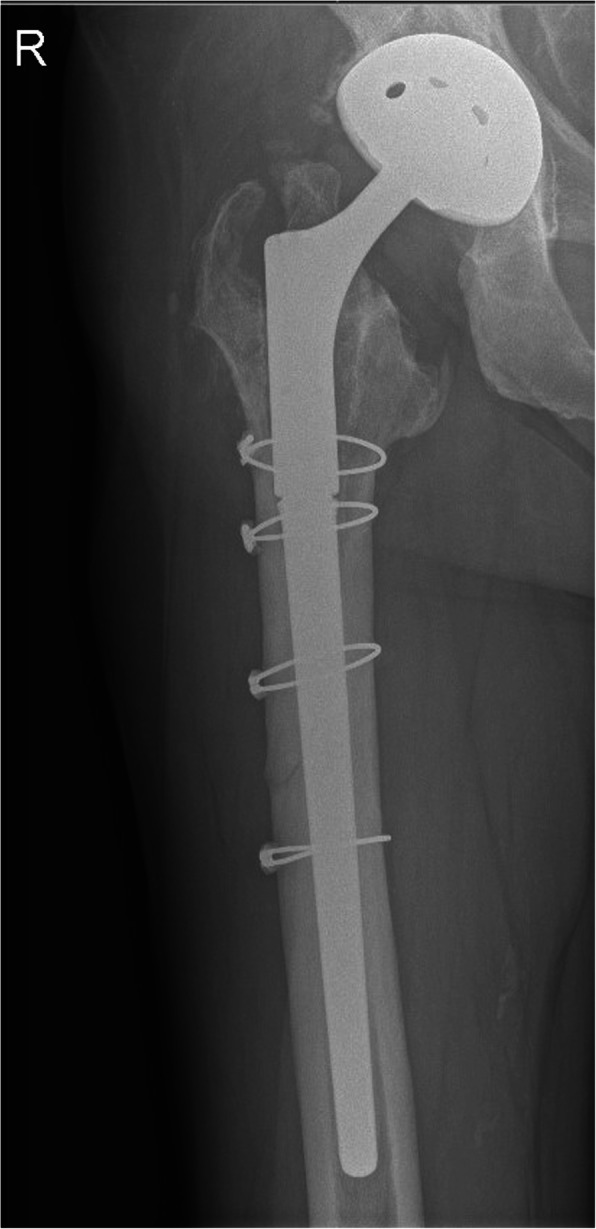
X-ray of the right hip 14 months after last revision
